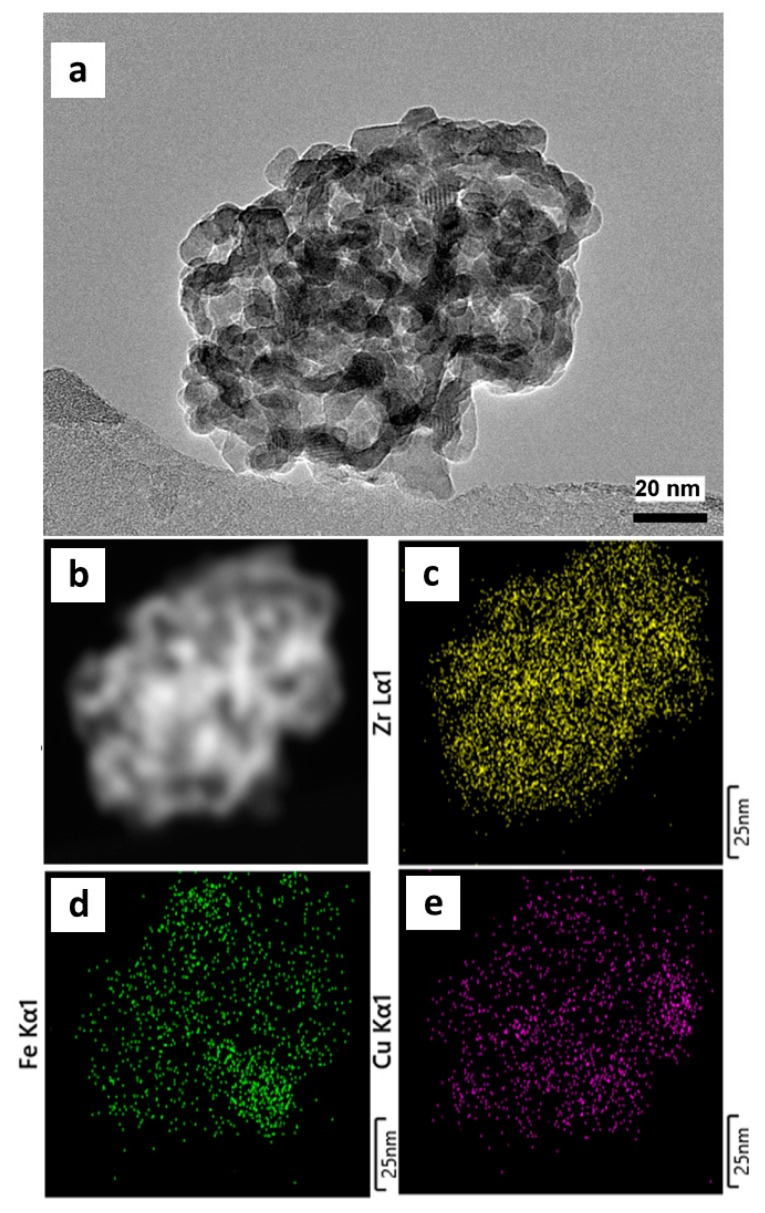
TEM images of Cu_16_Fe_7_/Zr catalyst: (a) selected area for the energy-dispersive X-ray (EDX) mapping in bright field; (b) selected area for the EDX mapping in dark field. Elementary mapping: (c) zirconium (yellow); (d) iron (green); (e) copper (magenta).
